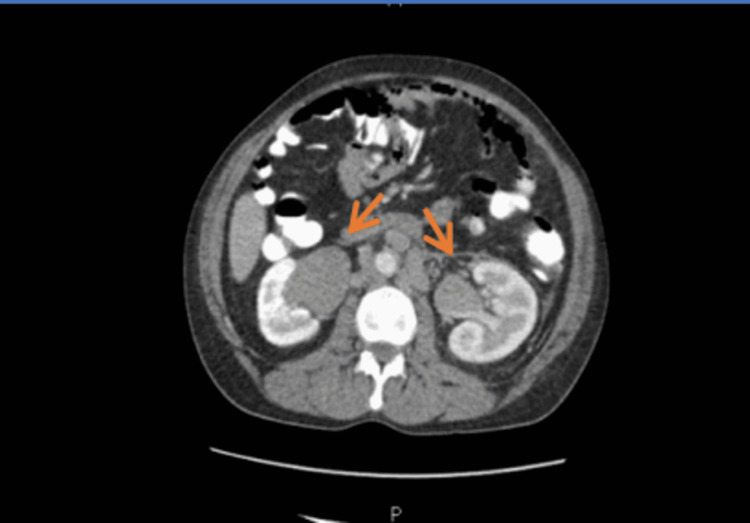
CT abdomen showing bilateral lymphadenopathy with hydroureteronephrosis (arrows). CT: computed tomography
